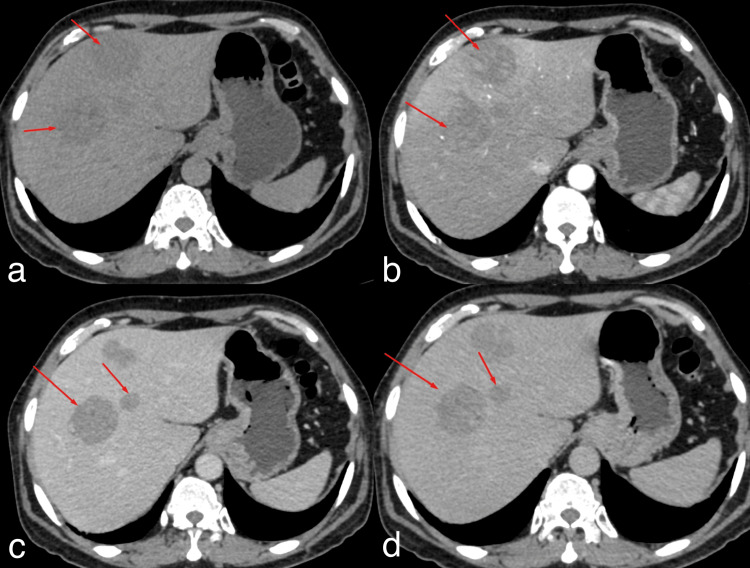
CECT of the abdomen. Contrast-enhanced computed tomography (CECT) showing multiple (four to five) well-defined lobulated heterogeneously hypodense lesions in the plain (a) and hypoenhancing lesions in the arterial phase (b) and venous phase (c) without appreciable washout in the delayed phase (d) in the right hepatic lobe, indicated by red arrows.
